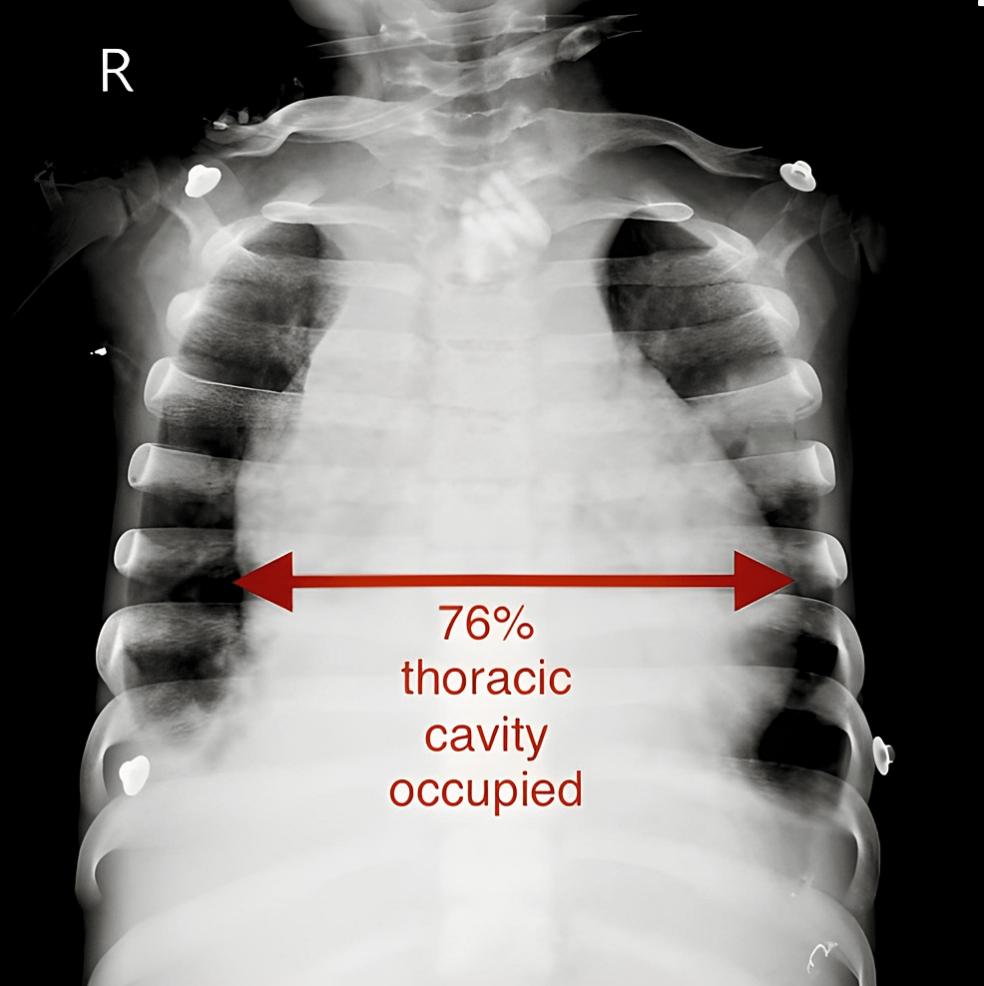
PA view of Chest X-ray (76% occupied by heart) PA=posterior-anterior
